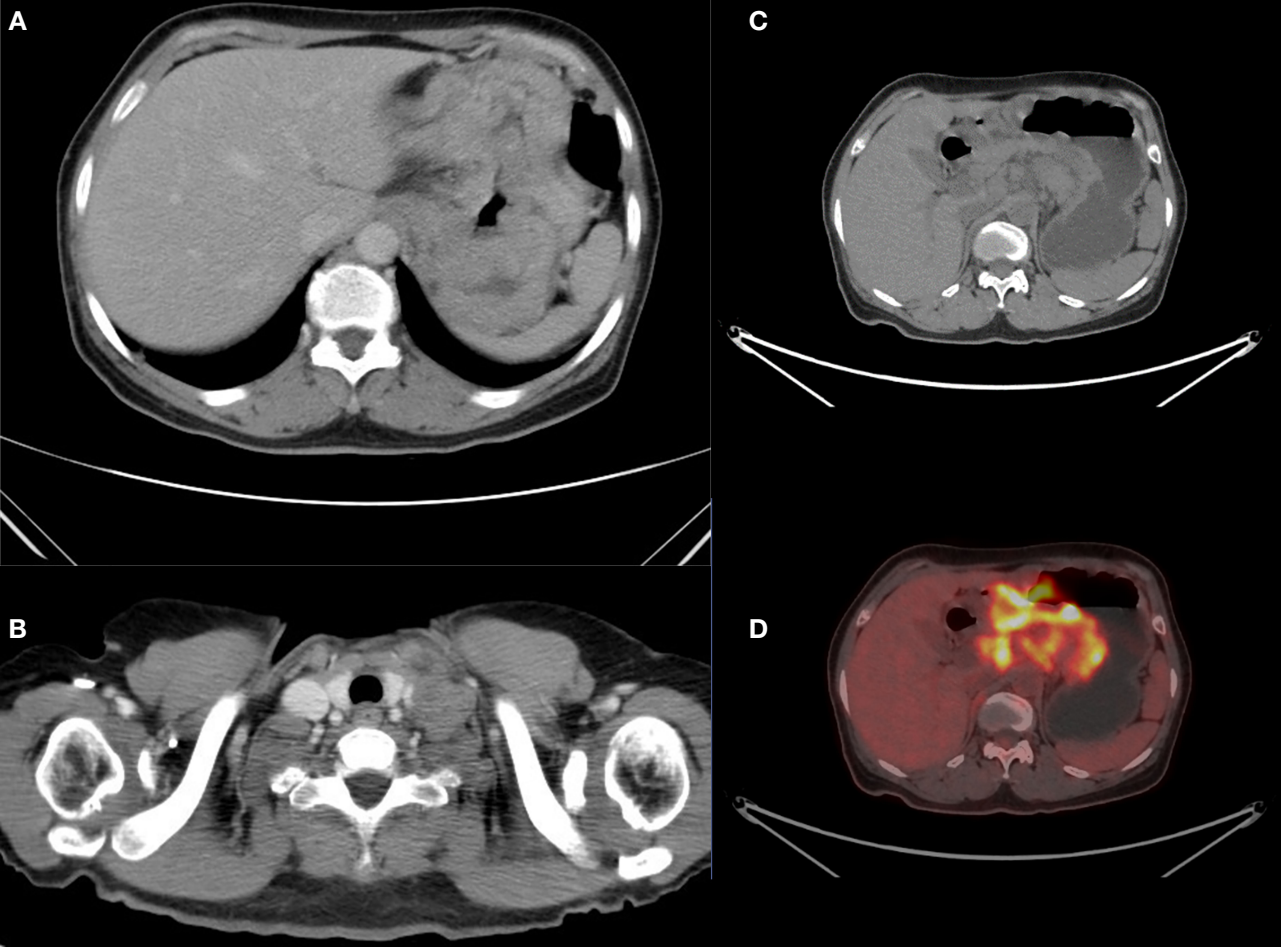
Computed tomography (CT) showed multiple enlarged lymphatic in the supraclavicular or infraclavicular fossa (A) and uneven enhancement and thickening in the gastric body fundus (B). The positron emission tomography (PET)-CT scan (C, D) revealed all lesions were metabolically active.
